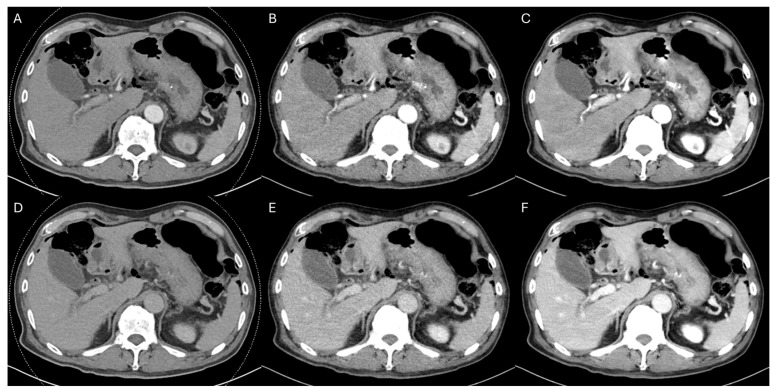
A 67-year-old man with extrahepatic cholangiocarcinoma who underwent an endoscopic ultrasound-guided hepaticogastrostomy. (A) Mixed image, arterial phase. (B) Conventional 50-keV VMI, arterial phase. (C) DLR-based 50-keV VMI, arterial phase. (D) Mixed image, portal venous phase. (E) Conventional 50-keV VMI, portal venous phase. (F) DLR-based 50-keV VMI, portal venous phase. A qualitative assessment of image quality, using a five-point Likert scale (higher scores indicate better quality), yielded the overall scores (Reviewer 1/Reviewer 2) (A) 3/2, (B) 4/3, (C) 5/4, (D) 3/3, (E) 4/3, and (F) 5/4, demonstrating higher overall image quality scores for DLR-VMI than for either the mixed image or conventional VMI in both phases. Both reviewers consistently reported improvements in noise, contrast, and sharpness during the arterial phase, and in noise and liver lesion conspicuity during the portal venous phases. Liver signal-to-noise ratios (SNRs) are provided for each panel, in the order displayed: arterial SNRs of 8.58, 6.19, and 9.17 for panels (A–C), and portal venous SNRs of 10.72, 8.44, and 14.10 for panels (D–F).
